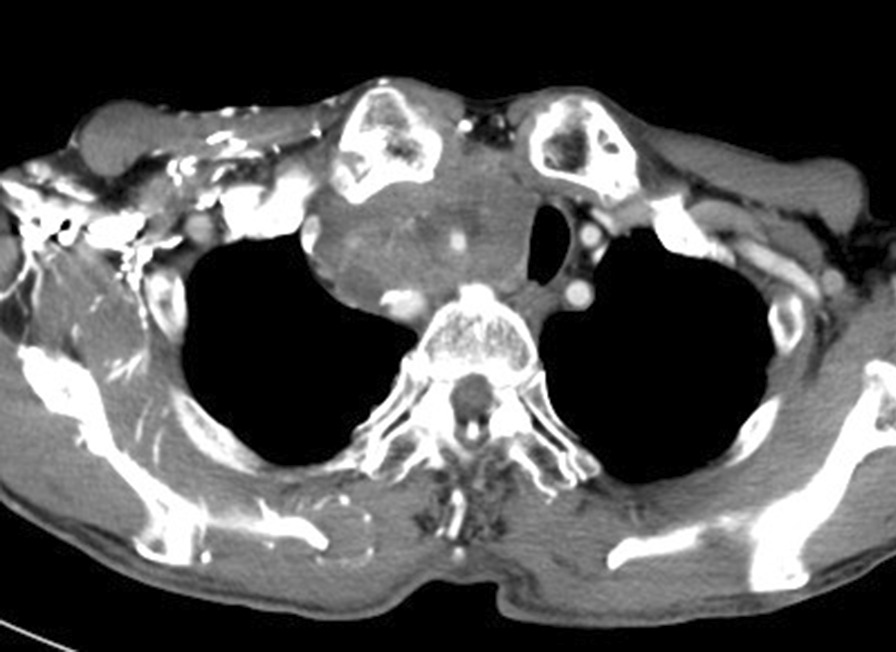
A CT scan showed a 70-mm right-sided infraclavicular lymph node metastasis. A left tracheal deviation was observed due to the tumor
